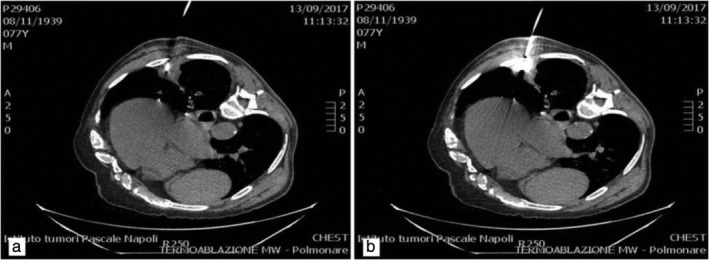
(a) Solitary fibrous tumor of the pleura. (b) Under multi‐detector computed tomography (MDCT) MW guidance, a needle was placed in the very center of the lesion.
